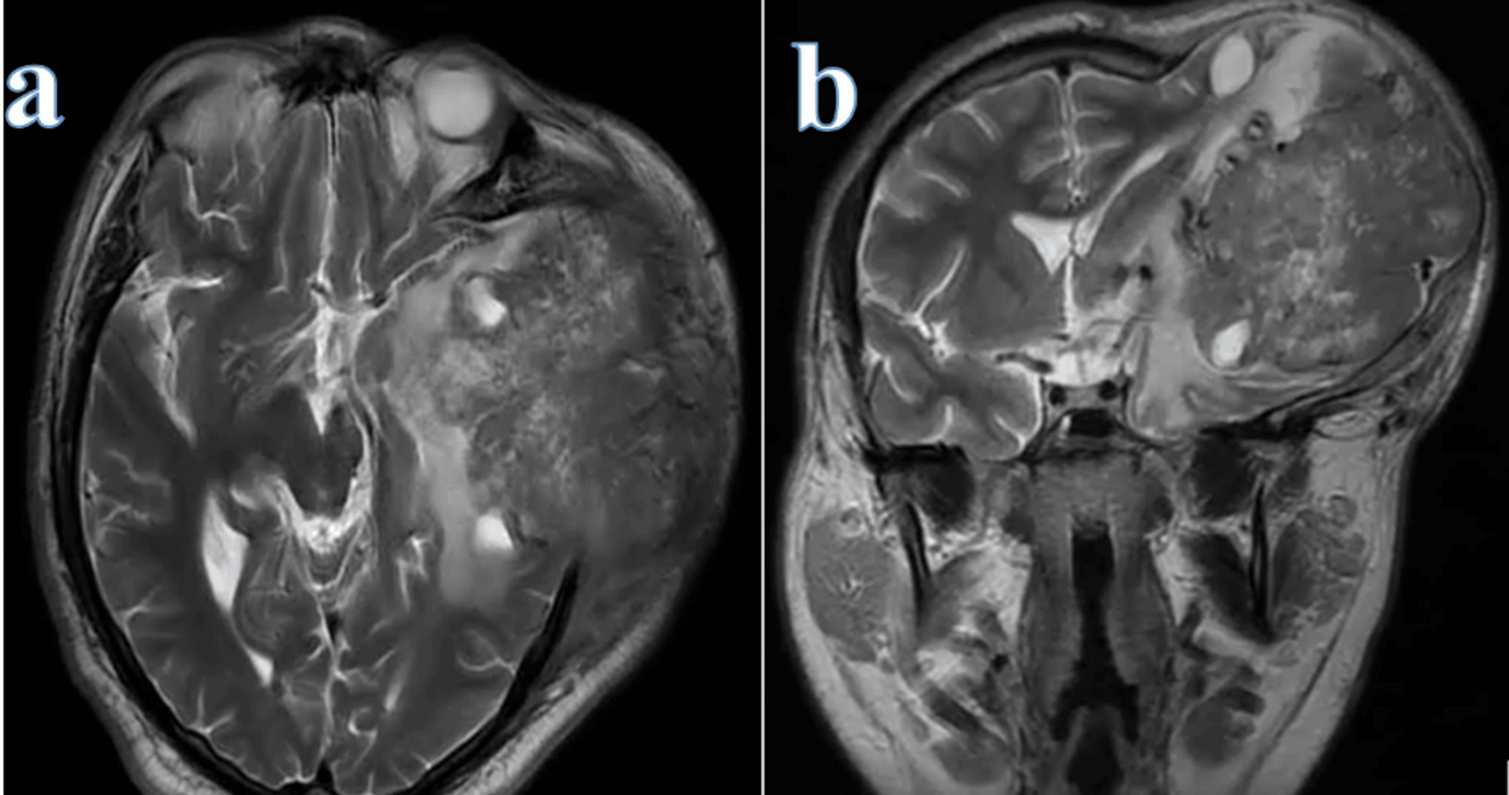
Preoperative MRI, 2019 T2-weighted MRI in axial (a) and coronal (b) sections showing a heterogeneous left-sided lesion with irregular margins, causing mass effect with midline shift, partial collapse of the ipsilateral ventricular system, and transcalvarial herniation.
